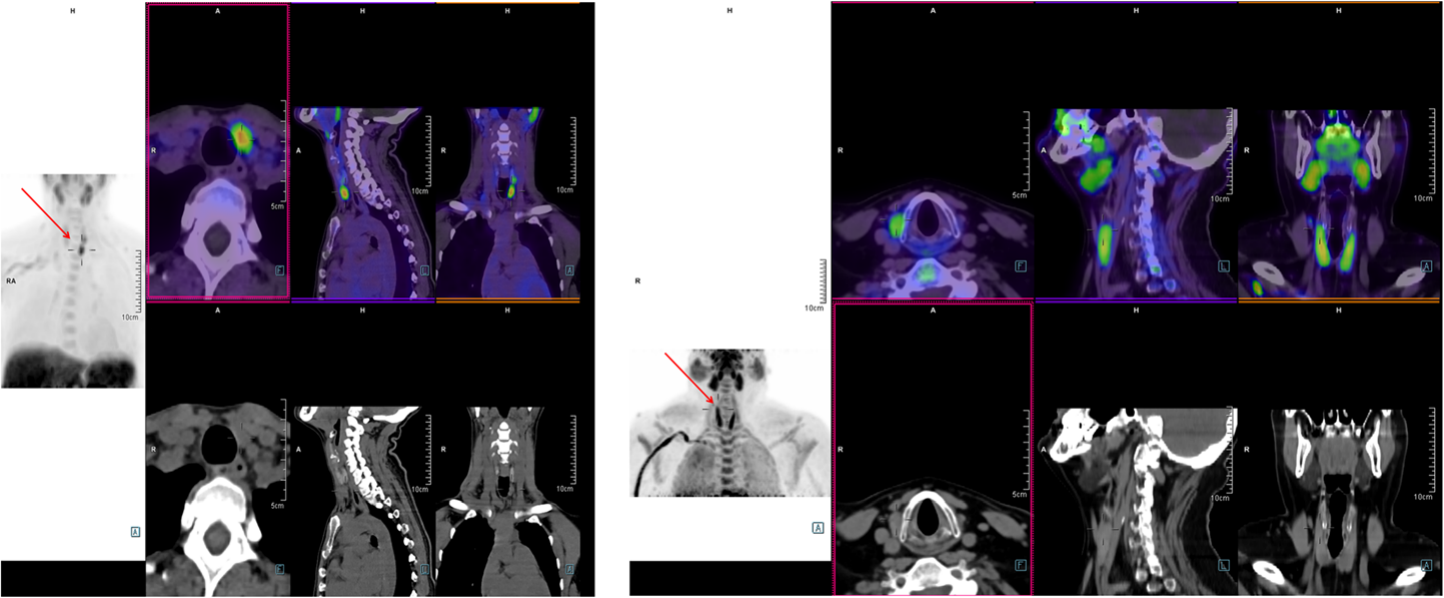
Two very different ^11^C-Choline PET/CT images. ^11^C-Choline positron emission tomography/computed tomography (PET/CT). Arrows mark the suspected hyperfunctioning parathyroid gland. Left: Image deemed of ‘high certainty’ by all three readers. Right: Image deemed of ‘low certainty’ by the expert reader (no images were deemed of low quality by all three readers). In the image to the right, choline uptake in the thyroid and salivary glands is clearly seen
